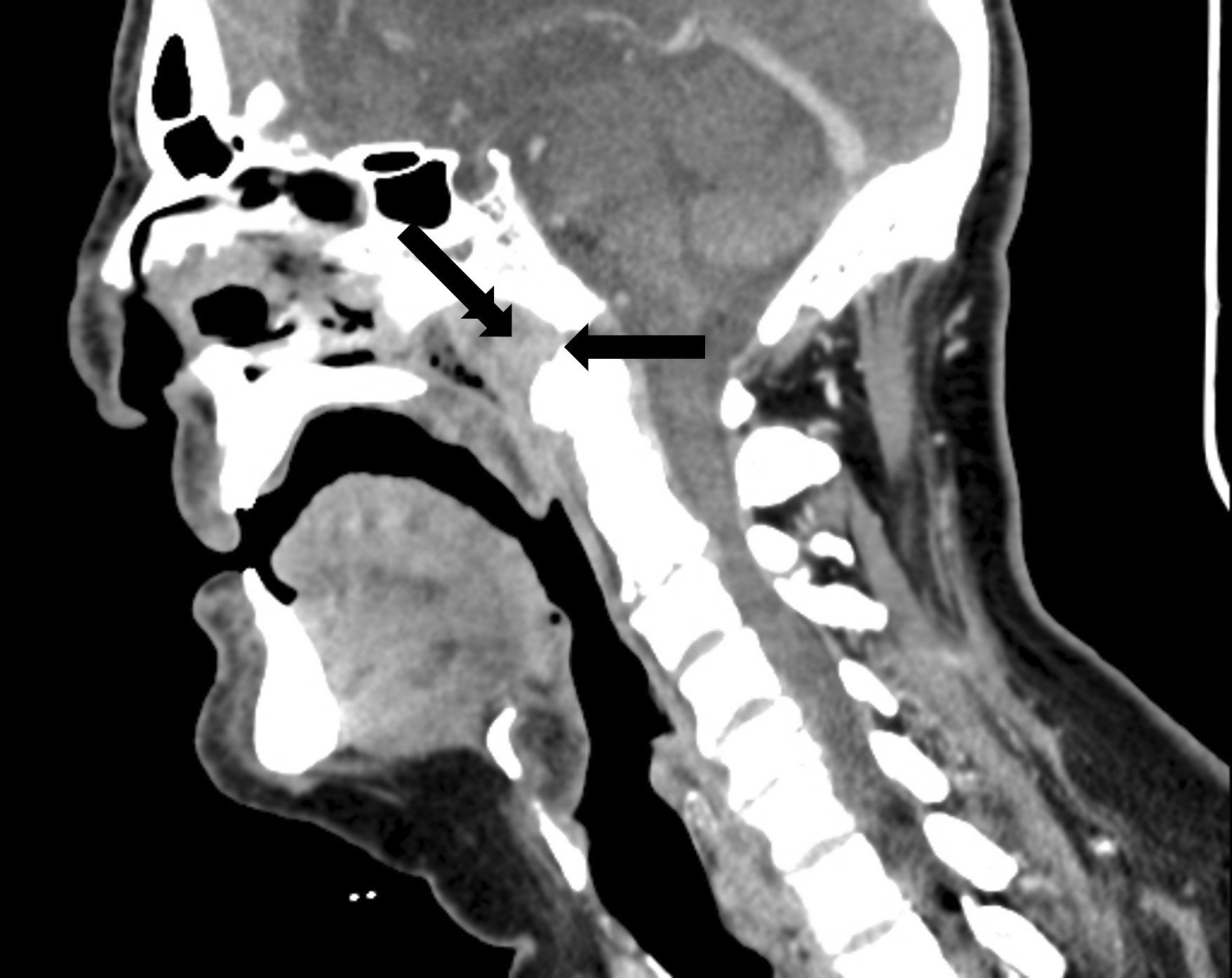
Sagittal CT scan showing partial clival erosion (simple arrow) with moderate soft tissue contrast enhancement (double arrow).
